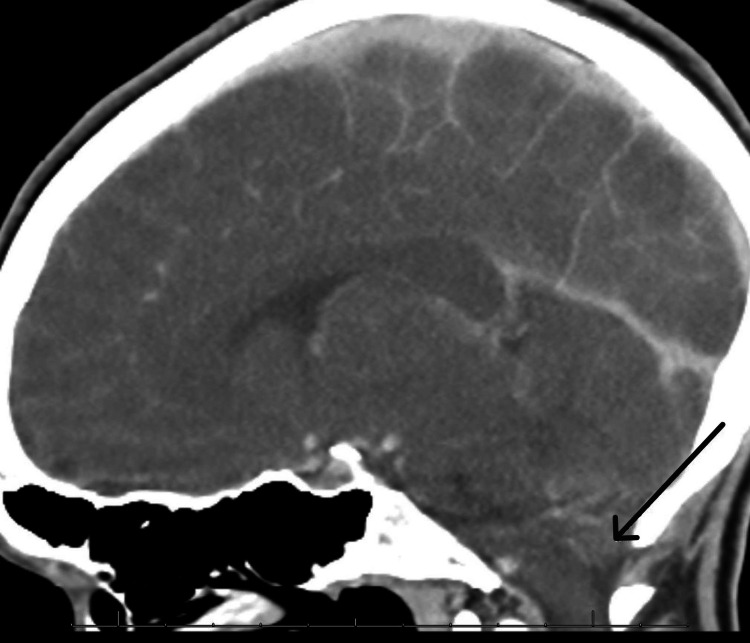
Sagittal CT of the brain after lumbar puncture The image is showing cerebral edema and downward displacement of the brainstem (black arrow).
